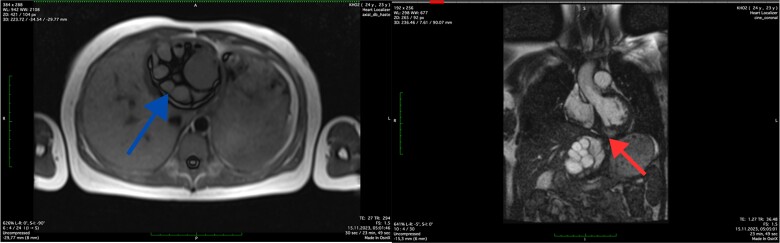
Axial MRI scan of the liver (blue arrow) and coronal MRI scan of the left ventricle (red arrow) showing the cystic lesions.
